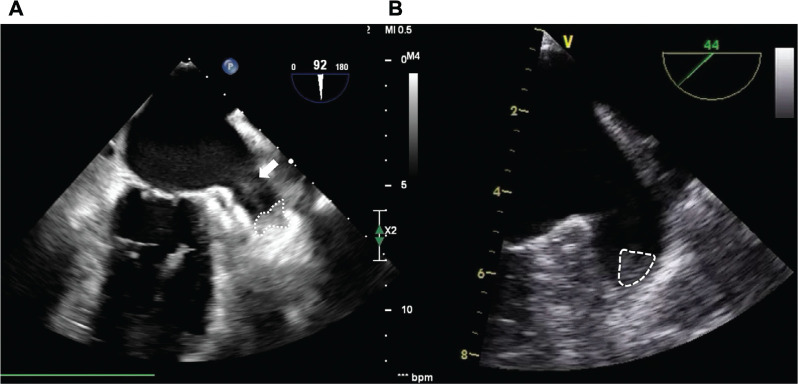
A: Forty-four months after empirical left atrial appendage (LAA) isolation, transesophageal echocardiography (TEE) revealed the presence of a thrombus (white dashed contour) within the distal part of the LAA, and spontaneous echocontrast (SEC) in the proximal part of the LAA (white arrow). B: Following a 6-week period, a subsequent TEE was performed, and the LAA thrombus remained persistent, while the SEC had resolved (white dashed contour).
